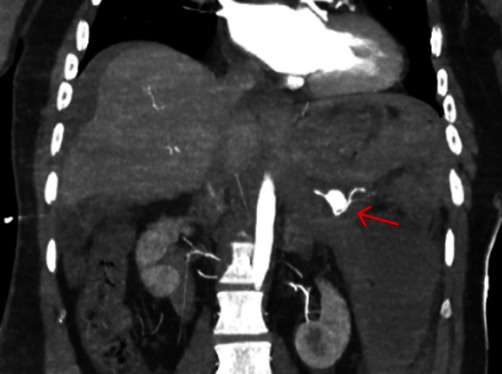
Large volume hemoperitoneum with active contrast extravasation from splenic artery on abdominal CT angiogram.
